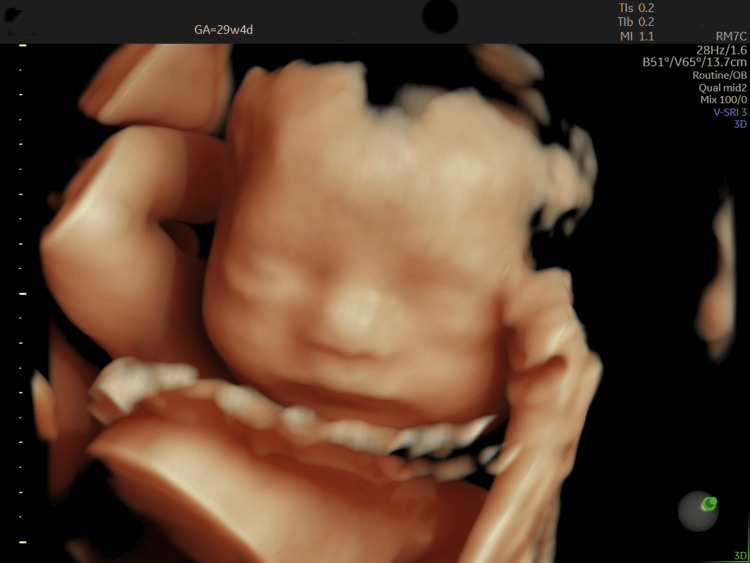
3D ultrasound depicting grossly normal craniofacial structure despite a 2D finding of a short nasal bone. Image taken by the author.
